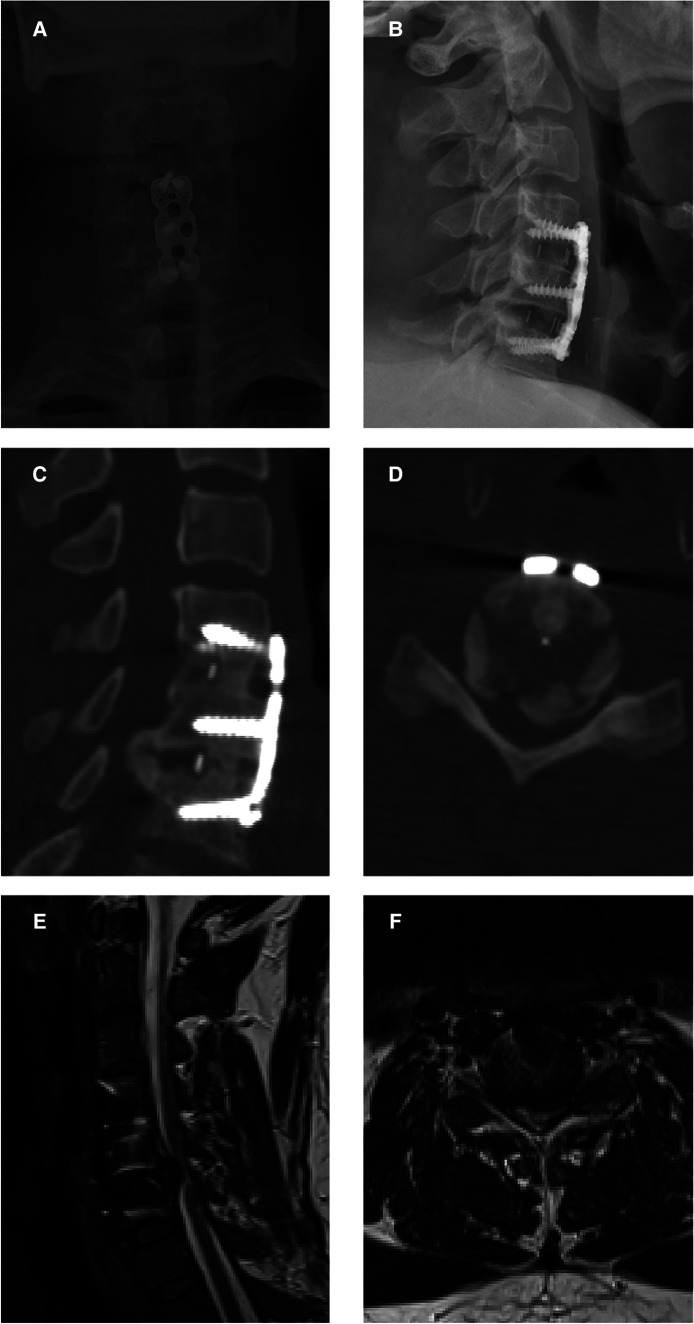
Preoperative images before open-door laminoplasty. (A,B) Preoperative x-ray images showing a large posterior cervical osteophyte at the level of C5/6. (C) A preoperative sagittal CT image showing a large heterotopic bone achieved from the posterior side of the C4 inferior end plate to the posterior side of the C5 superior end plate. (D) A transverse CT image revealing evident cervical stenosis and anterior spinal cord compression due to ossification. (E,F) Preoperative MR images showing the narrow space of the spinal canal and a T2-weighted hyperintense intramedullary signal at the C5/6 level.
